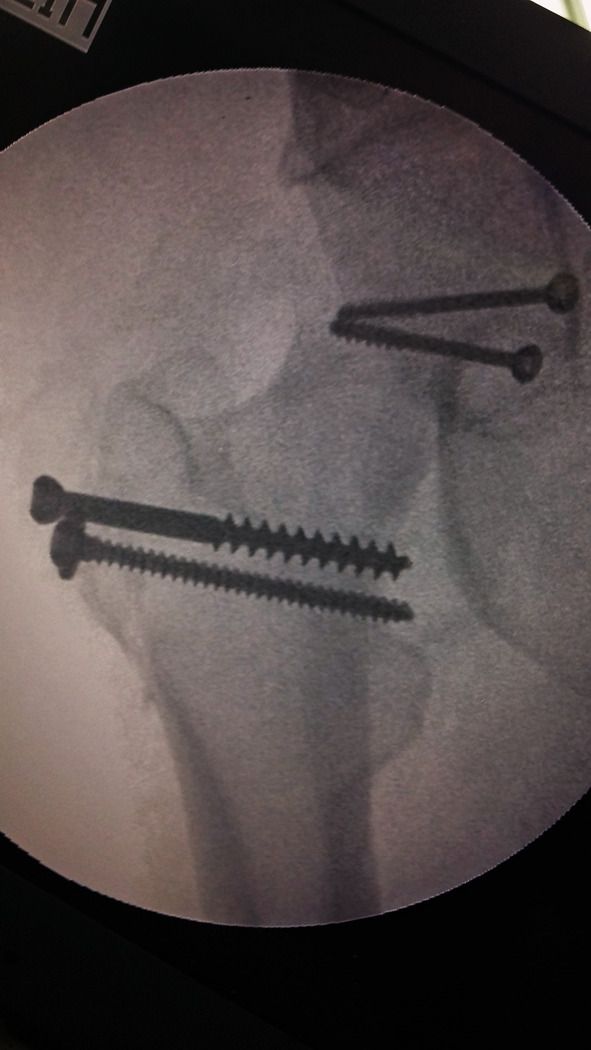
Immediate postoperative plain radiograph showing femoral head fixation
